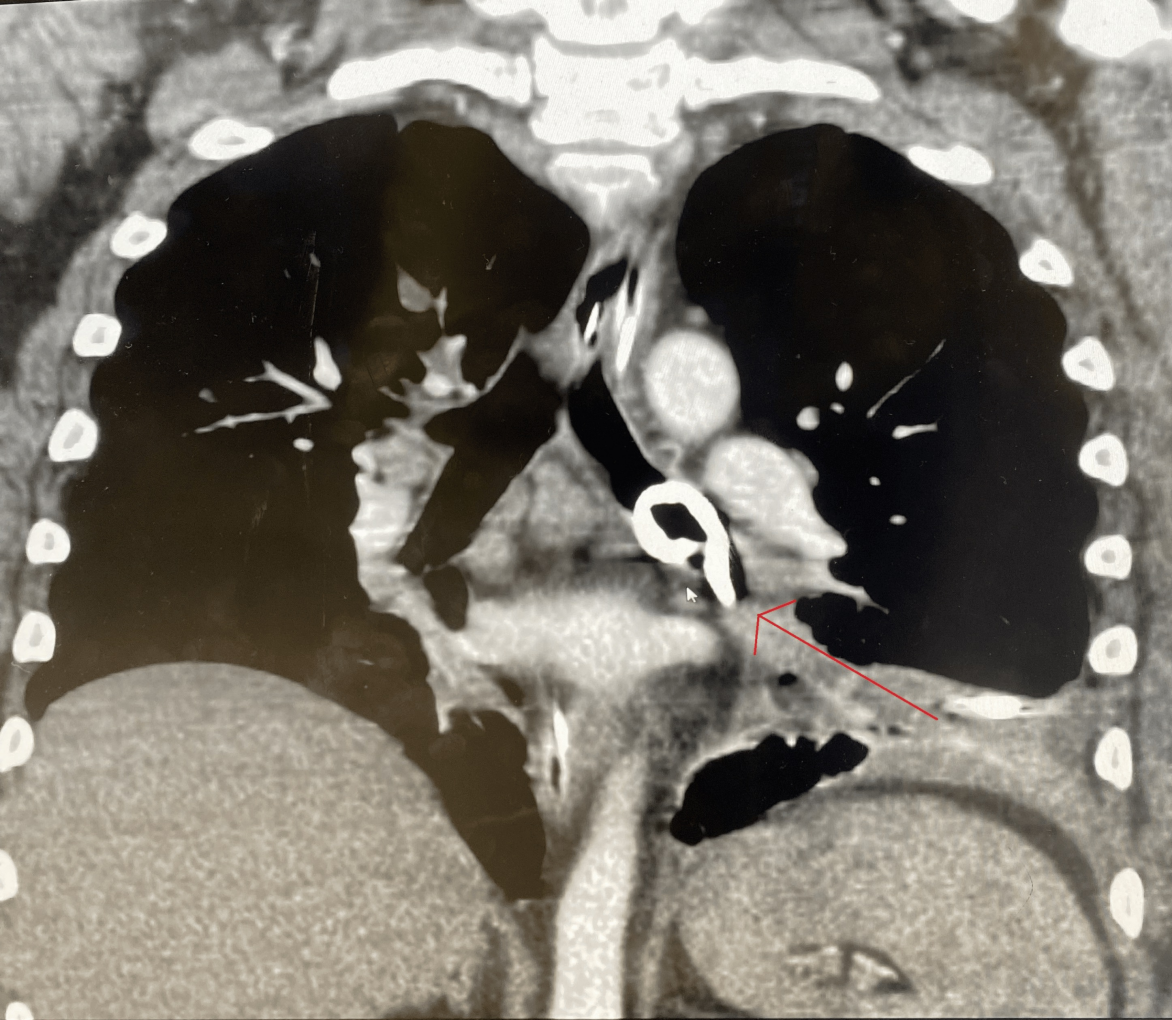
Day 7 CT angiography (coronal view) showing the pleural catheter in the left mainstem bronchus
